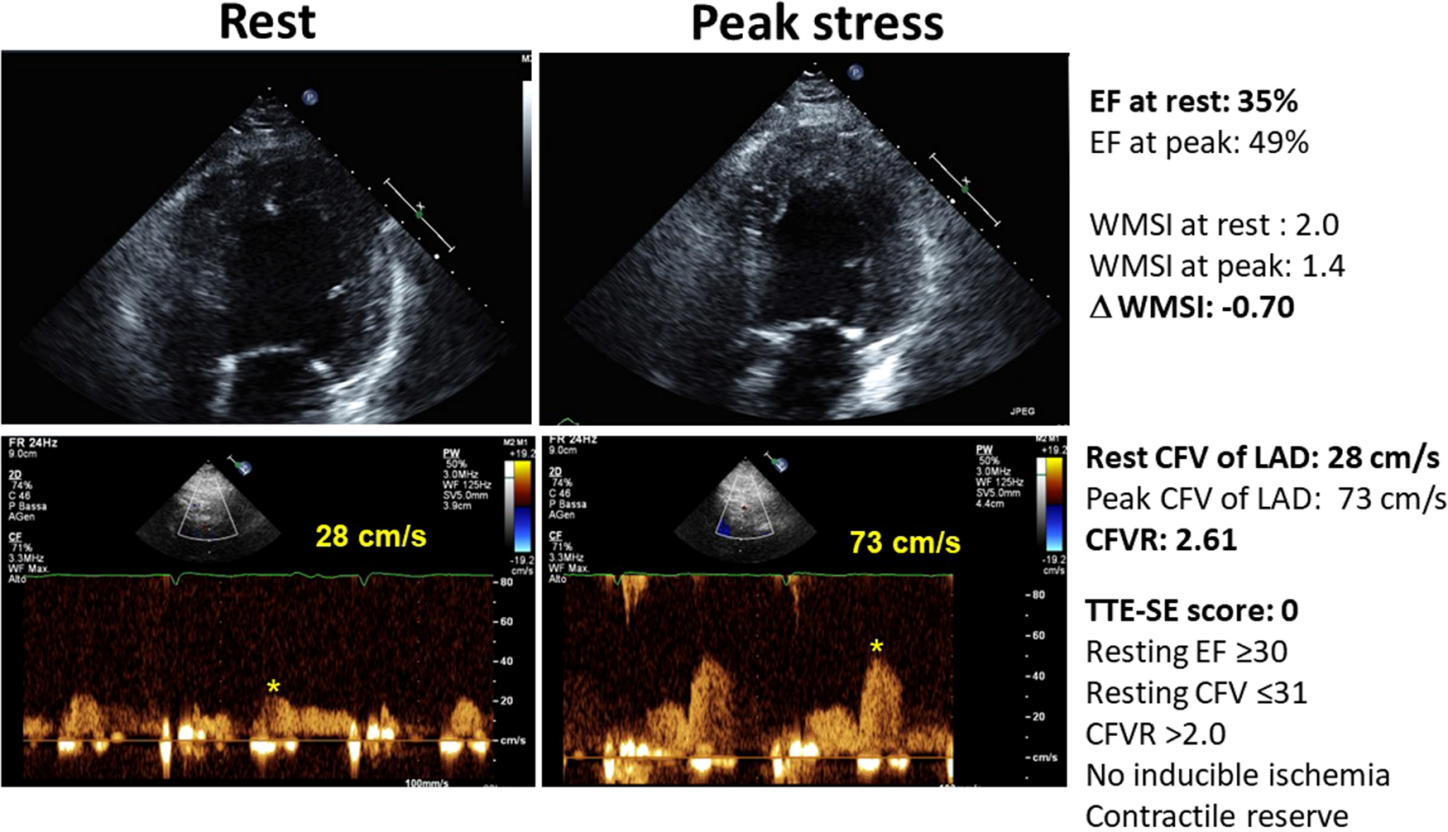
The normal pattern of normal resting and peak flow velocity with dipyridamole, with normal reduction of LV end-systolic volumes and regional wall motion improvement during stress. Upper panel, color-Doppler signal; middle panel, pulsed-wave Doppler signal; lower panel: end-systolic frame of the LV.
